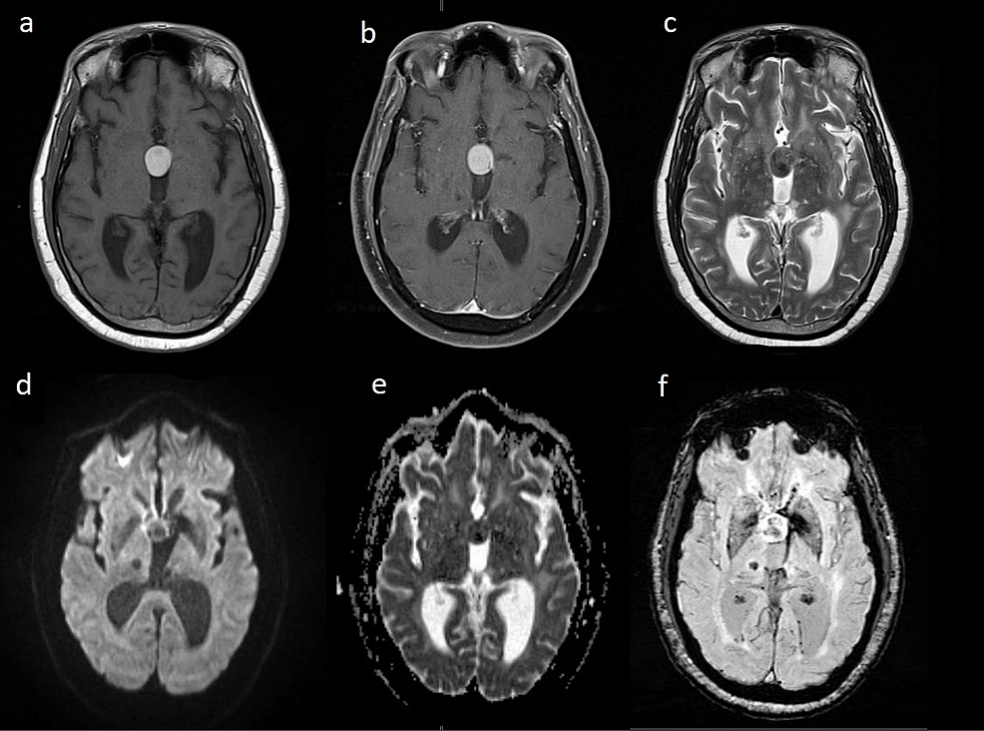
MRI brain, November 27, 2020, further characterizing third ventricular mass (a) T1-weighted image showing hyperintense third ventricular lesion; (b) T1 contrast-enhanced image showing hyperintense third ventricular lesion; (c) T2-weighted image showing heterogeneously hypointense third ventricular lesions; (d) DWI demonstrating no diffusion restriction within the third ventricular lesion; (e) no ADC correlate within the third ventricular lesion; (f) SWI demonstrating no hemorrhage or calcifications within the third ventricular lesion. DWI: diffusion-weighted imaging; ADC: apparent diffusion coefficient; SWI: susceptibility-weighted imaging
